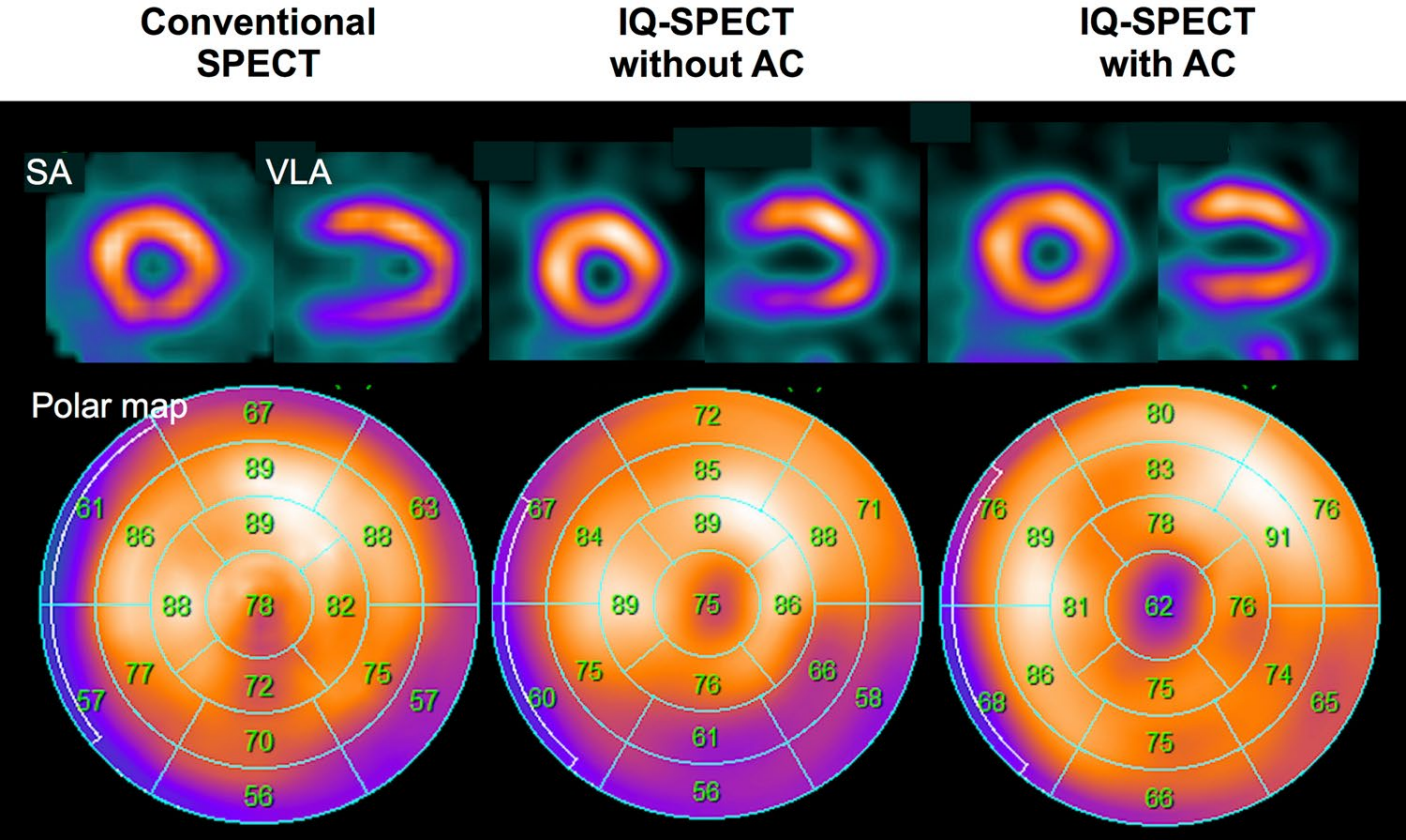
SPECT images of a normal subject with three SPECT conditions. Conventional MPI (left), IQ·SPECT without (mid) and with attenuation correction (AC) (right) are shown. *SA* short-axis, *VLA* vertical long-axis
